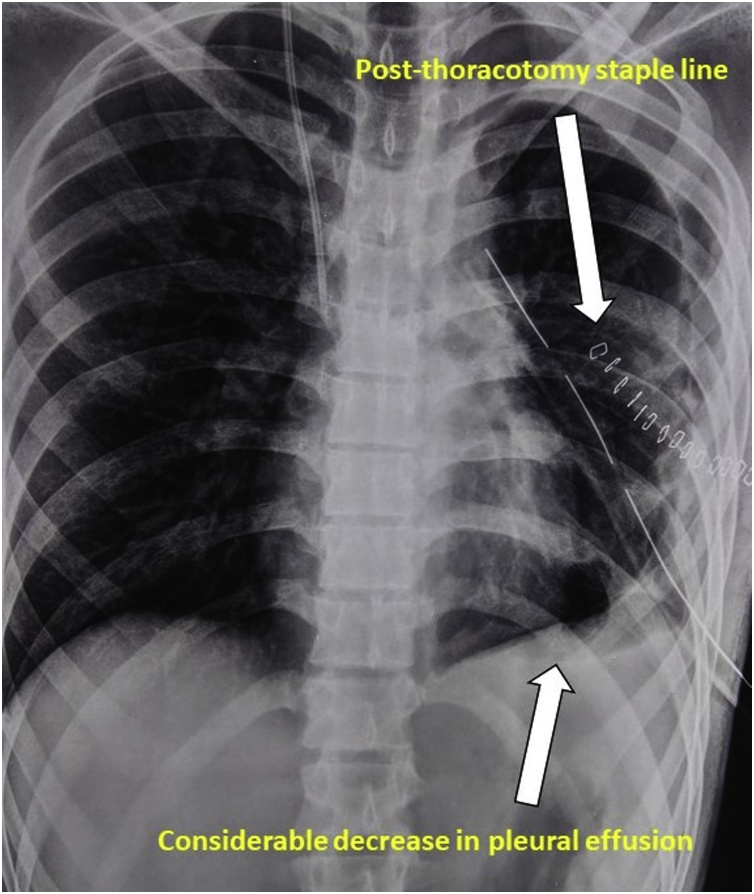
Chest radiography done post mini-thoracotomy showed "considerable decrease in pleural effusion”.
